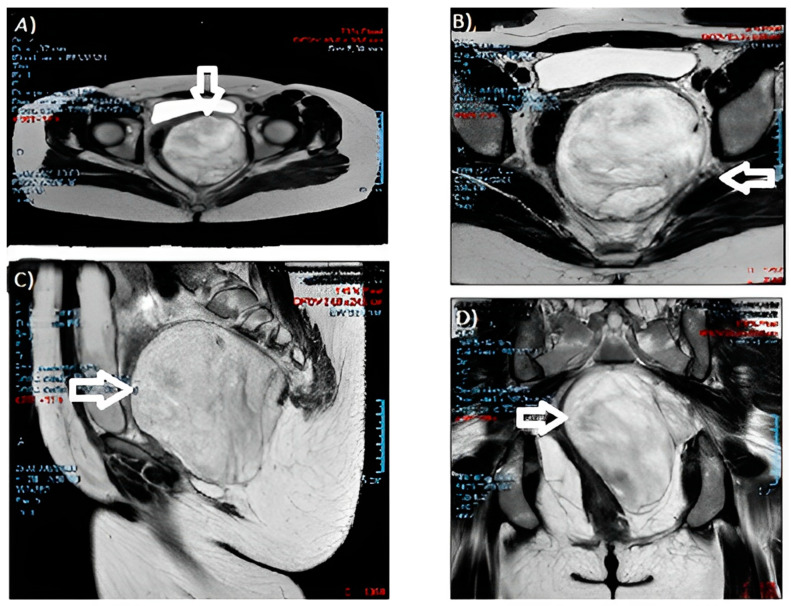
MRI images of the angiomyxoma: (A,B) cross-sections; (C) sagittal section; and (D) coronal section. (The arrow indicates the neoformation).
